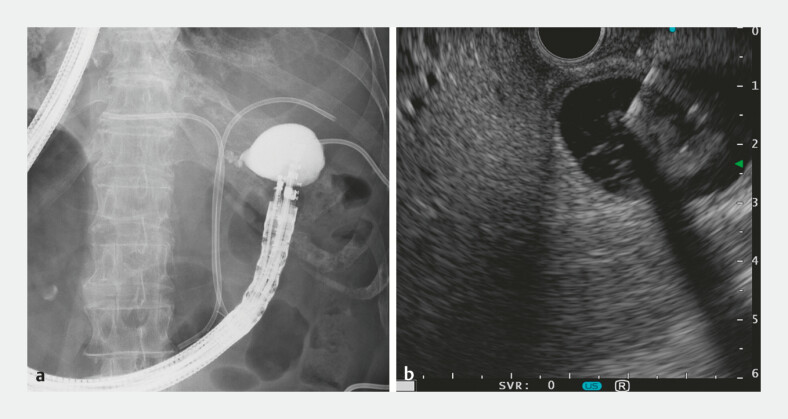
Endoscopic ultrasound-guided gallbladder drainage in situs inversus totalis.
